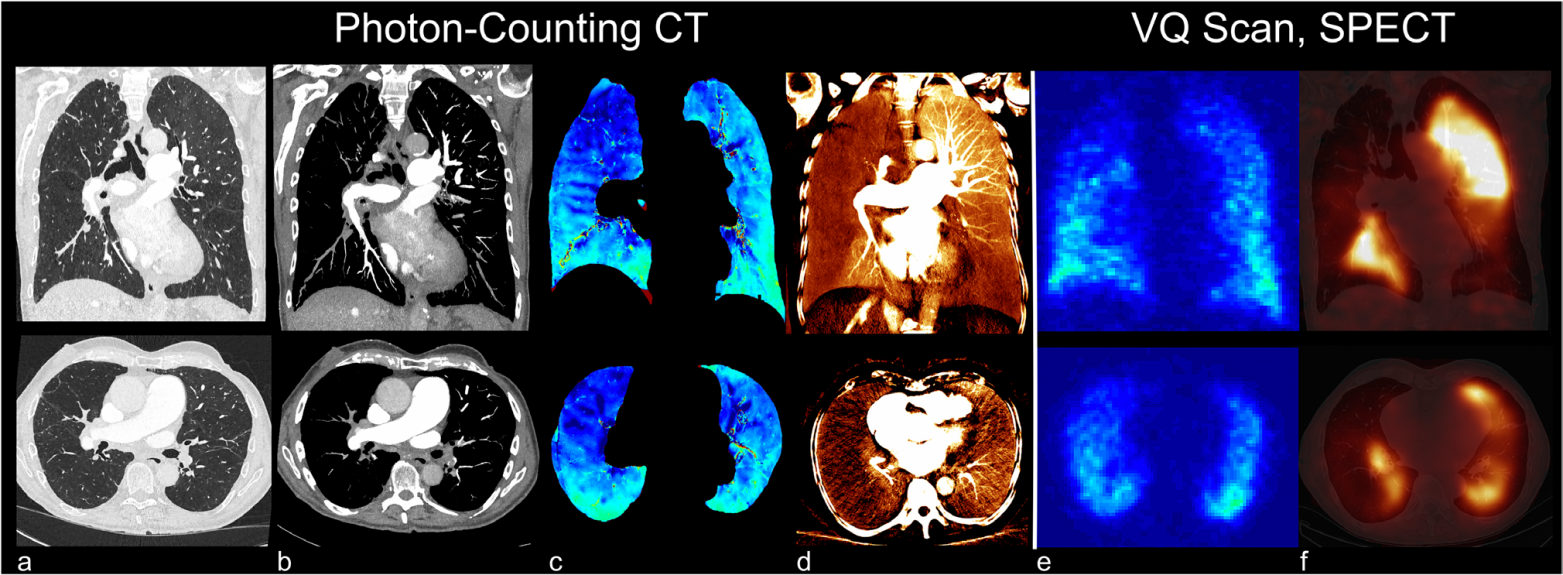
Photon‐counting CT images of a patient with chronic thromboembolic hypertension (CTEPH). Photon‐counting CT allows a comprehensive assessment of the lung structure and vasculature (a, b) and function (ventilation and perfusion: c, d) with high spatial resolution. CT ventilation and perfusion (c, d) show high agreement with VQ‐SPECT (e, f). VQ‐SPECT, ventilation/perfusion single‐photon emission computed tomography.
